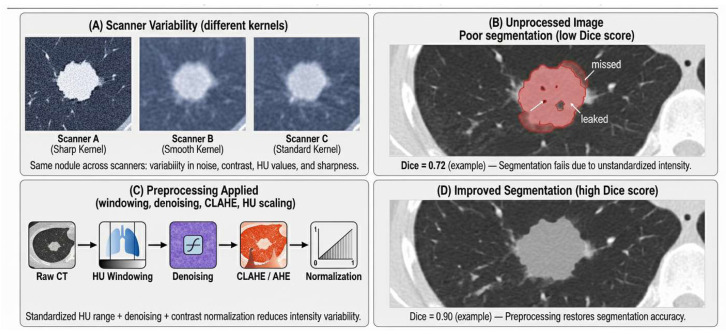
Impact of CT variability on lung-nodule segmentation and the role of preprocessing. (A) Scanner Variability with different kernels; (B) Unprocessed Image with Poor Segmentation (low dice score); (C) Preprocessing Applied with different techniques (Windowing, denoising, CLAHE, HU scaling); (D) Improved Segmentation (high dice score).
